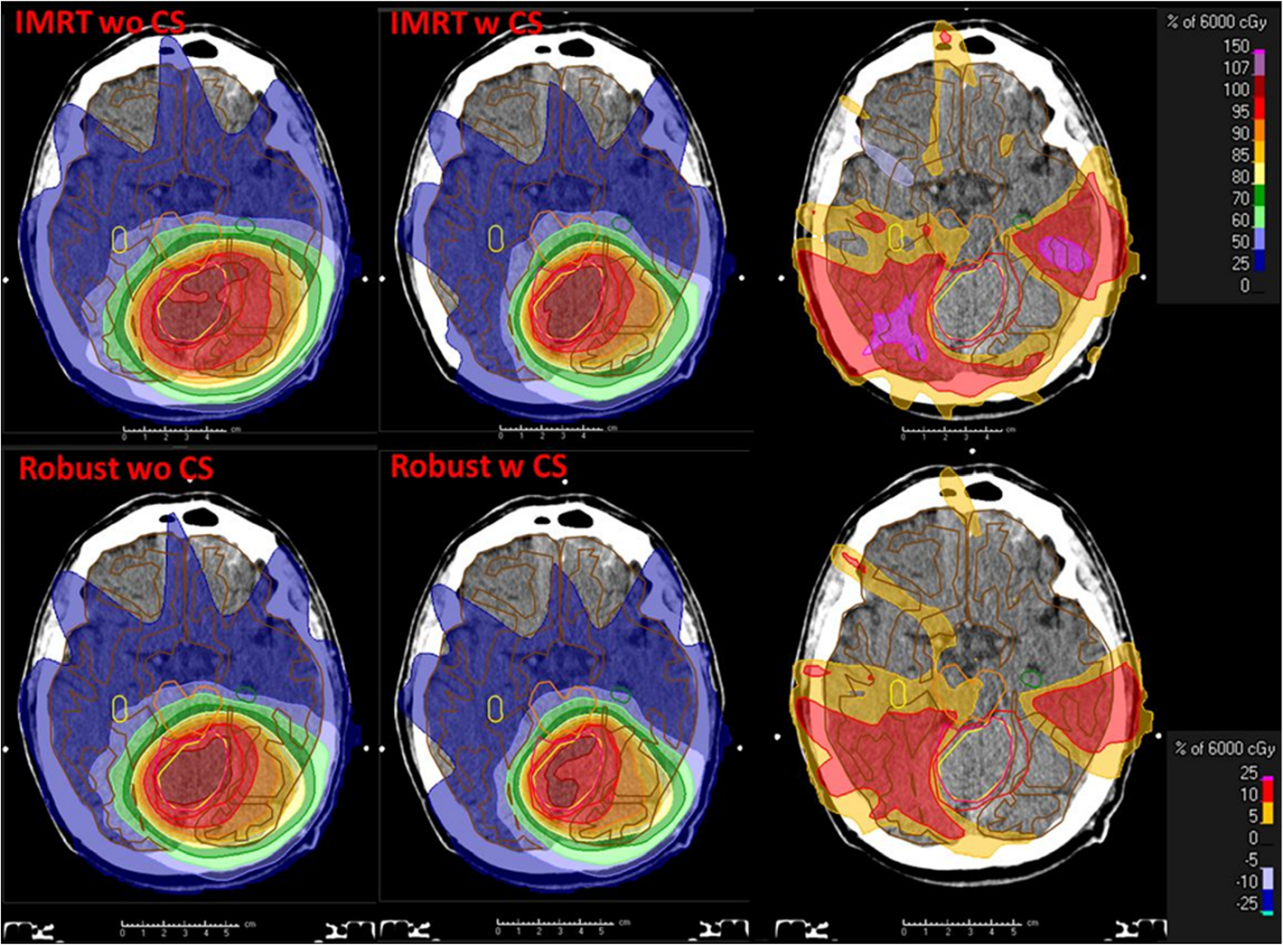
IMRT and robust dose distributions for patient A on a representative CT slice. Left column: IMRT/ robust plan without cortex sparing (wo CS). Middle column: IMRT/ robust plan with cortex sparing (w CS). Right column: dose difference (plan wo CS minus plan w CS). The dose difference shows the potential of cortical sparing of up to 25% (purple area) for this slice. Red and yellow areas indicate a dose reduction of 10%, and 5%, respectively
